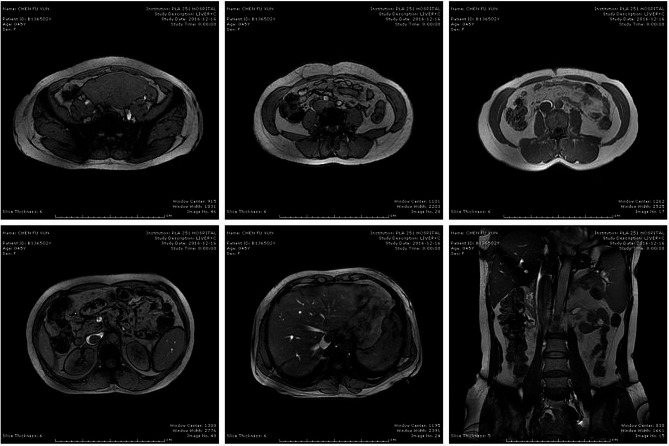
Enhanced Magnetic Resonance Image of abdomen revealed obviously enlarged uterine and bilateral ovaries, with no clear boundary. The tumor extended through venae iliaca interna to inferior vena cava
